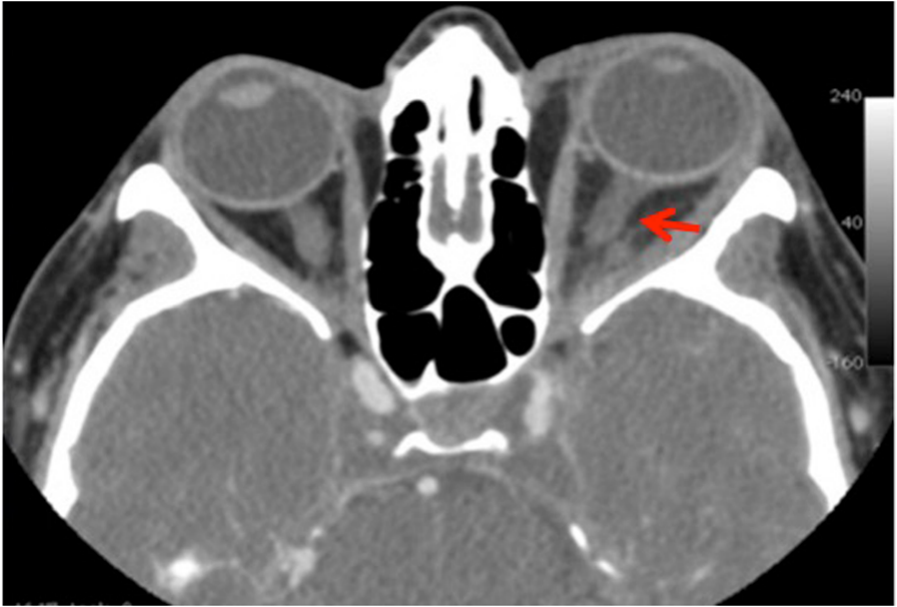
Contrast-enhanced computed tomography. Distended superior ophthalmic vein on the *left side* (*arrow*), with periorbital swelling
